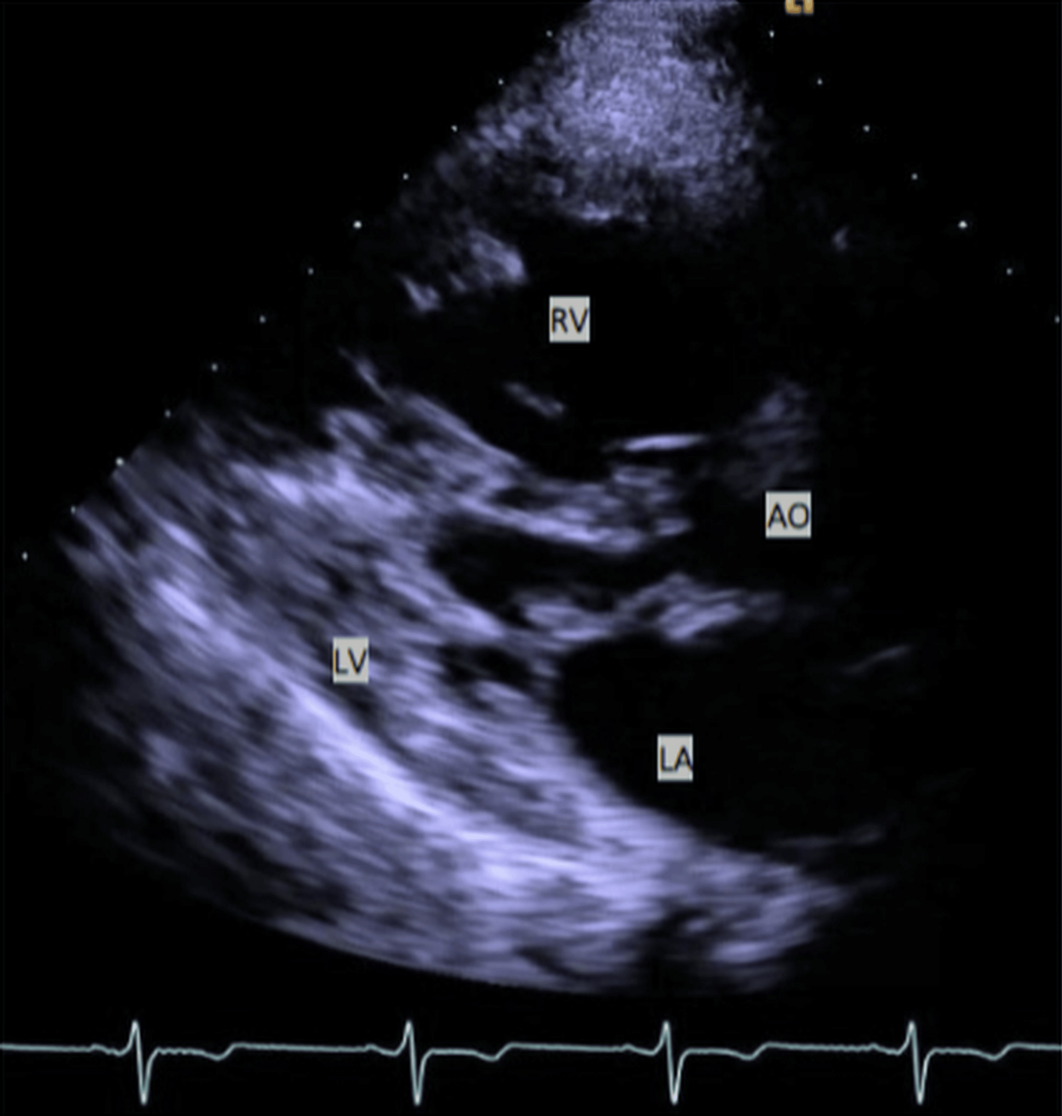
Parasternal long axis TTE view of the heart in a patient with HLHS. The image features the markedly hypoplastic LV and the systemic RV. TTE, Transthoracic echocardiogram; HLHS, Hypoplastic left heart syndrome; RV, Right ventricle; LV, Left ventricle; LA, Left atrium; AO, Aorta
